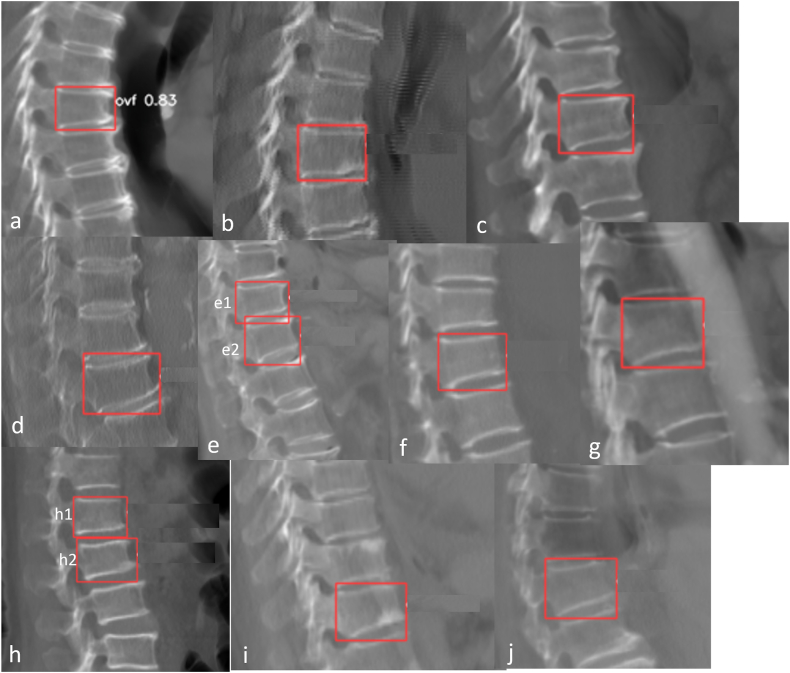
CSR CT images of false positive readings recorded in 12 vertebrae in 10 cases (A: VF probability is denoted as 0.83). Most of these vertebrae had slightly wedged shape but were not labeled as VF by the reading radiologists. The image in E is affected with two adjacent VFs (e1, e2); They could mostly be easily evaluated by a radiologist reader as being of no significance or a false positive reading.
